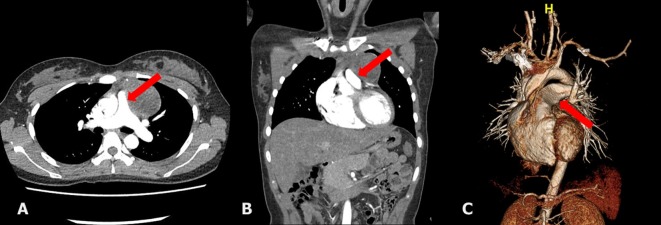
The chest CT showing a lobulated, inhomogeneous mass containing fat component in the mediastinum, compressing main pulmonary trunk (arrow), and no definite invasion to lung parenchyme (A, B, C).
